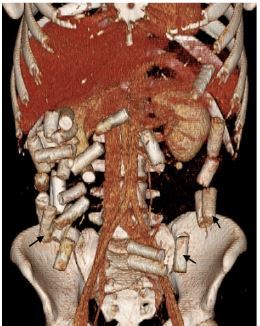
Computed tomography of the abdomen using three-dimensional reconstruction showing extensive tubular foreign bodies present throughout the colon (arrows).
